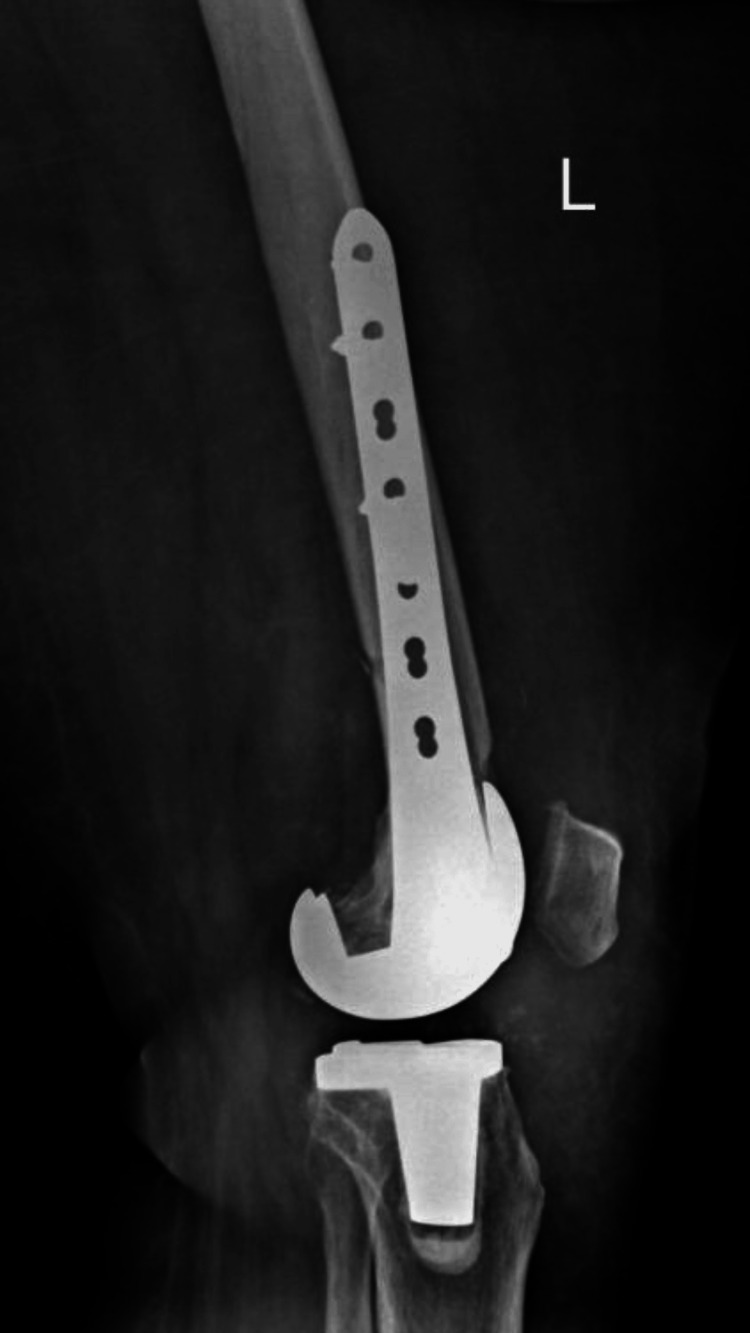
Lateral radiograph of the left femur demonstrating postoperative fixation of the periprosthetic distal femoral fracture using a locking plate system. The prosthetic components remain well aligned, and fixation appears stable without evidence of hardware failure.
